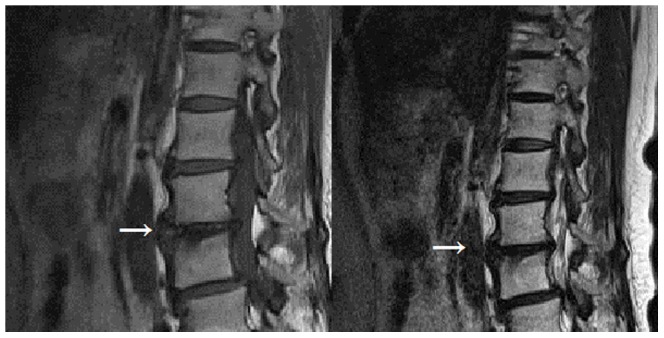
Modic type III changes (indicated by arrow) appear hypointense on. T1-weighted and hypointense, on T 2 weighted images.
